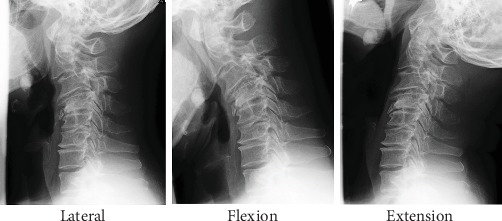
Preoperative X-ray. Axis slipped anteriorly by 7 mm. No change in slippage was observed in the flexion and extension positions.
